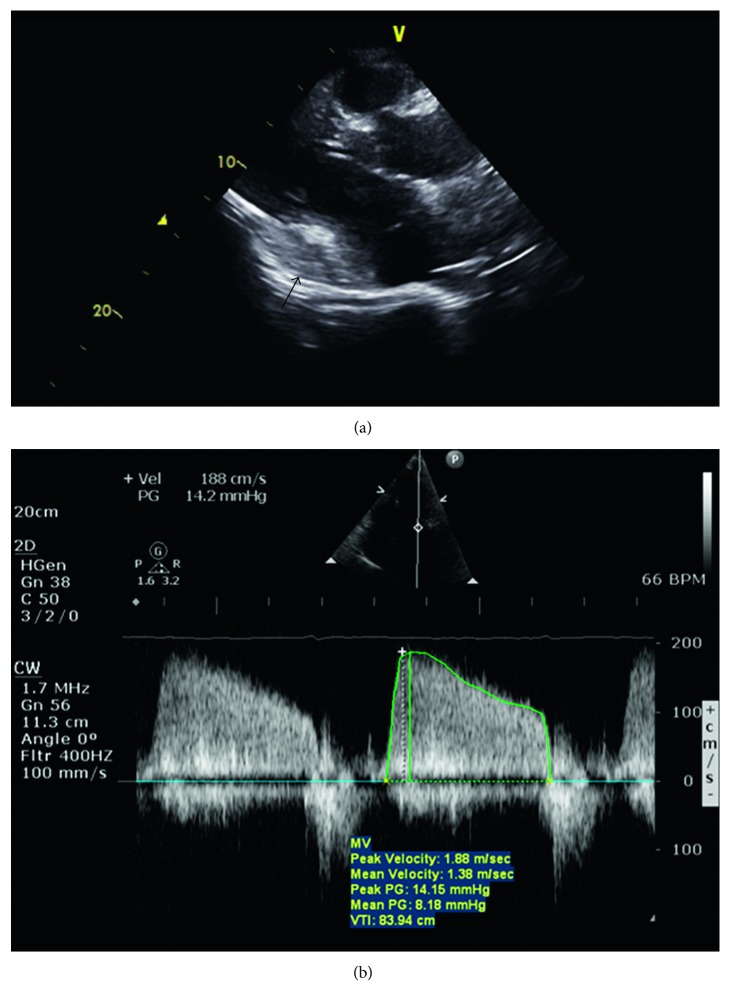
(a) Emergency transthoracic echocardiogram showing accumulation of hematoma around the coronary sinus and atrioventricular groove. (b) Pulse Doppler in the apical 4-chamber view across the mitral valve showing elevated mean pressure gradient of 8 mmHg, suggesting functional mitral stenosis secondary to compression of the left atrium and mitral valve apparatus.
